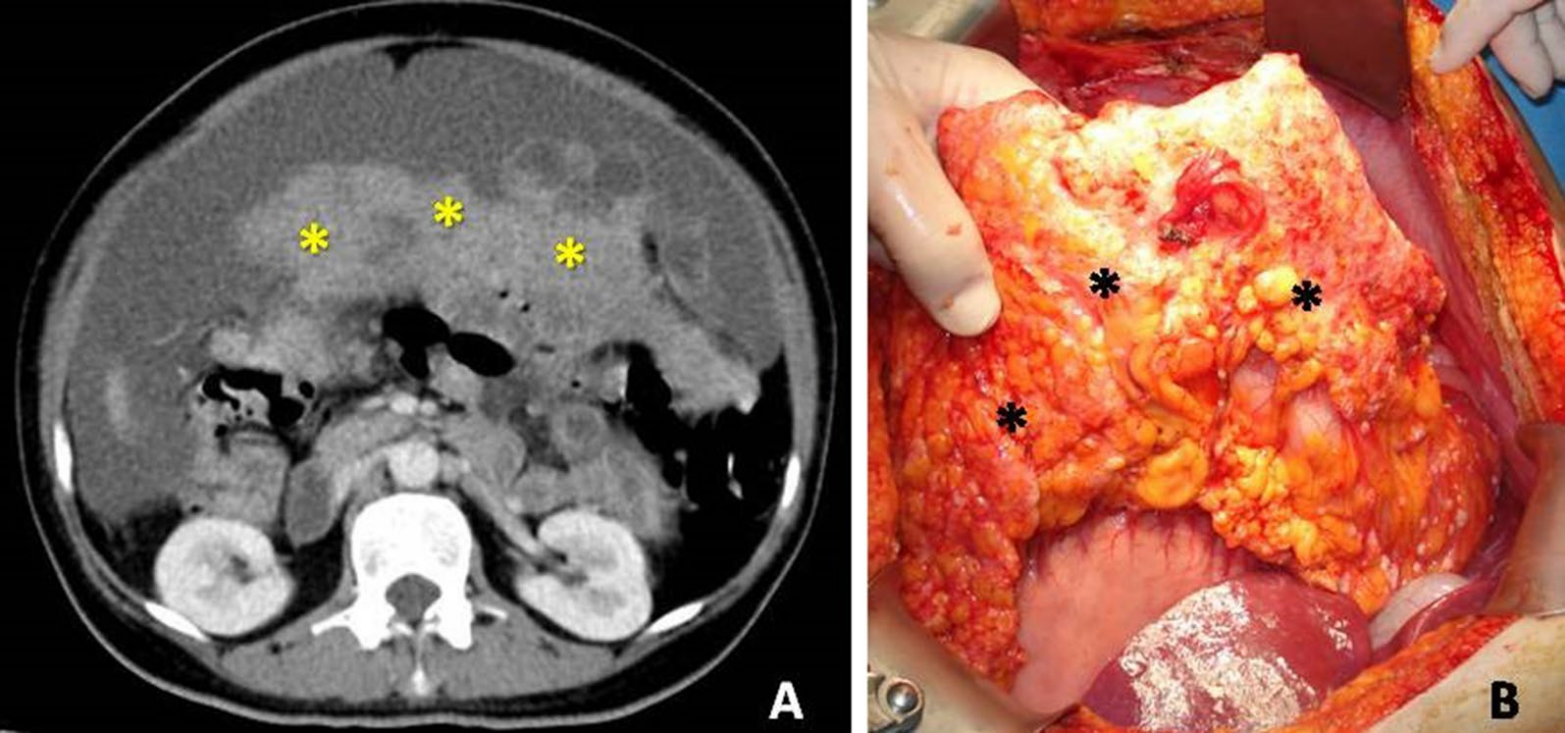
a Contrast-enhanced CT image reveals massive infiltration of the greater omentum—“omental cake” (asterisks). b At surgery extensive infiltration of the omentum is demonstrated (asterisks)
